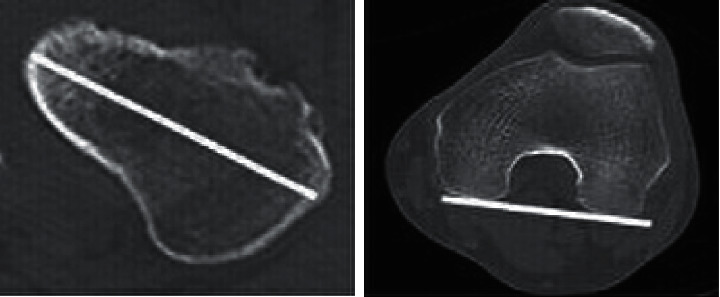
Femoral neck anteversion angle. The femoral neck anteversion angle is measured by identifying the femoral head-neck axis from the CT horizontal section slice just beneath the femoral head, with reference to the posterior condylar axis.
